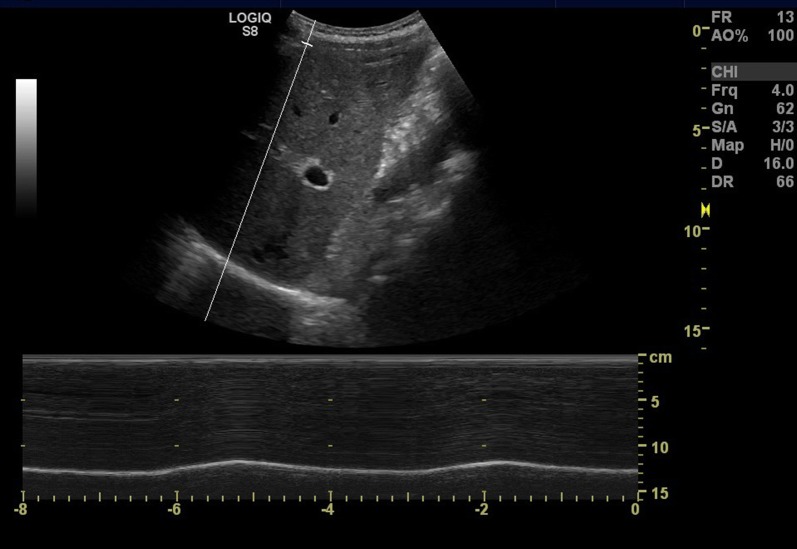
Subcostal ultrasound image in mid-clavicular line with M-mode measuring diaphragm excursion
